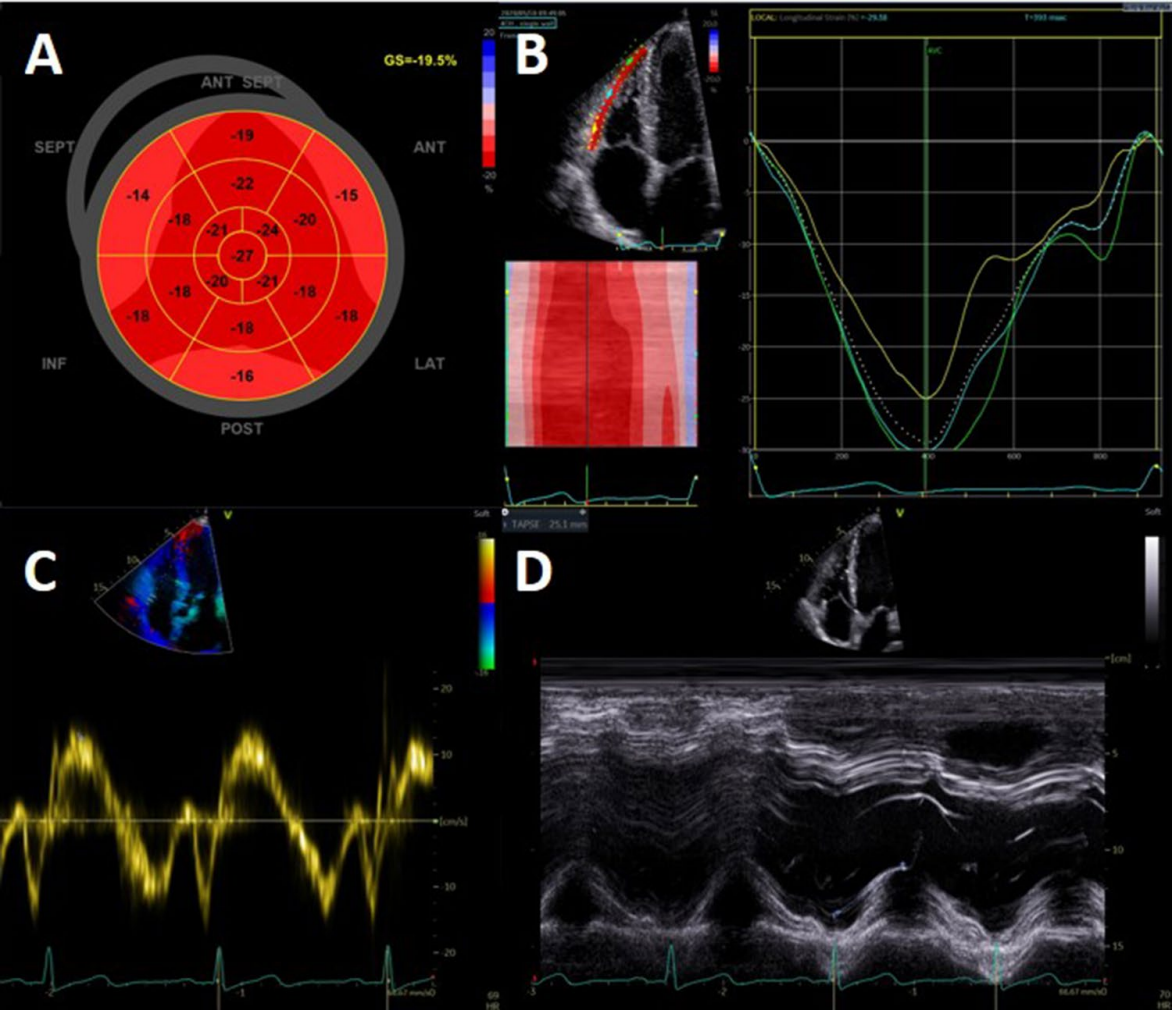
Case example of a COVID-19 patient with normal myocardial function. Case example of a 44-year old patient without prior medical history who had been admitted to the ICU for COVID-19 in the first wave and was on mechanical ventilation for 5 days. At the out-patient clinical he has persisting dyspnoea symptoms (NYHA II). Echocardiography shows no significant abnormalities. LVEF was 58% and LV GLS was normal (− 19%). Right ventricular function is preserved (TAPSE 25 mm,Sʹ 12 cm/sec, RV strain − 29)
